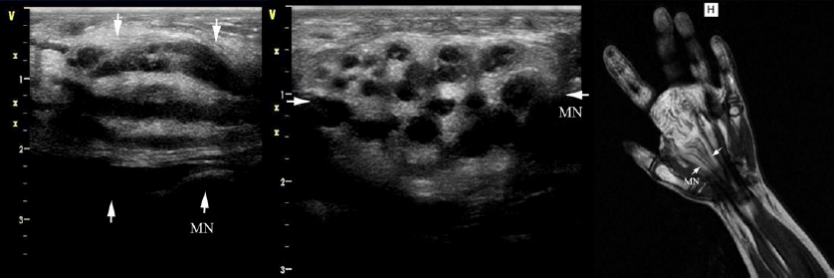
Ultrasonic and MRI images of fibrolipomatous hamartoma of the enlarged median nerve.
